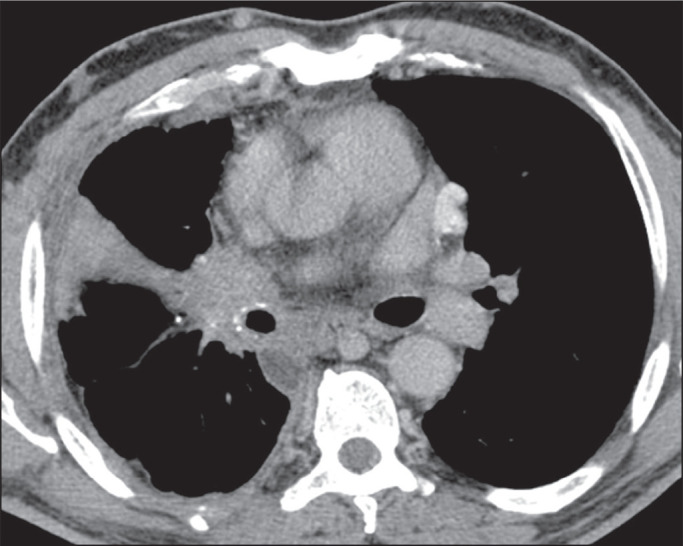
CT of a 51-year-old woman with fibrosing mediastinitis.
